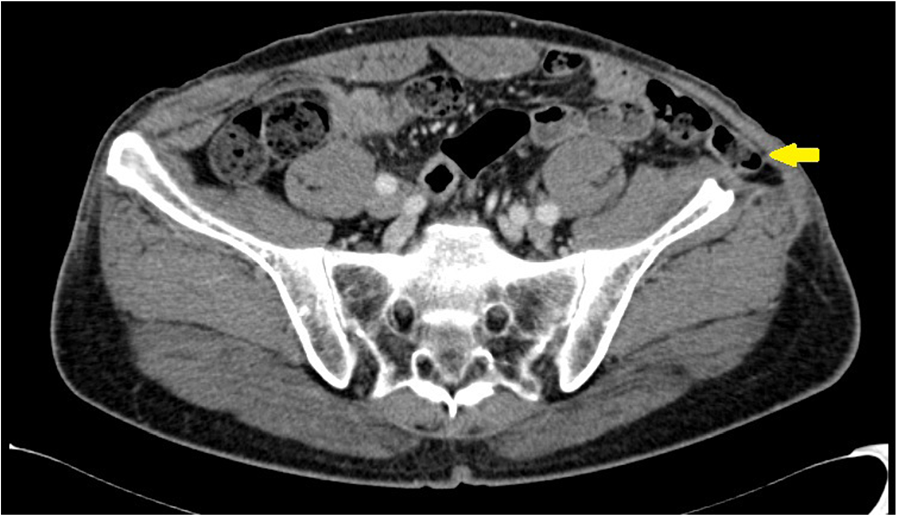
CT image (case 2). Herniation (*arrow*) can be observed through the bone defect caused by DCIA flap harvesting. There are no anatomical barriers to avoid herniation
